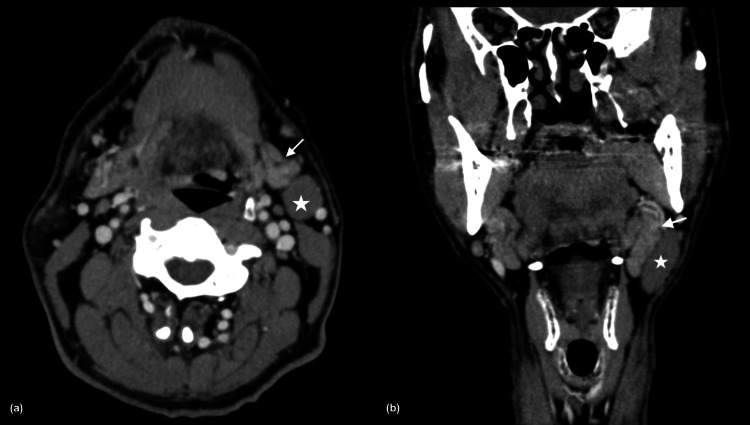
Contrast-enhanced computed tomography of the neck (a) A well-circumscribed, homogenous hypodense lesion (star) at the left lateral cervical region and a normal left submandibular gland (arrow); (b) a distinct separation from the tumor on the coronal.
